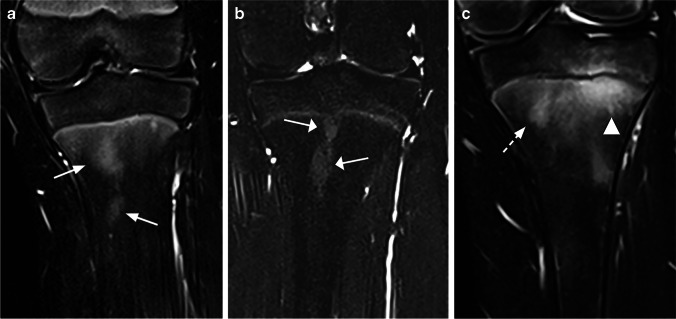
Coronal water-only Dixon T2-weighted magnetic resonance images in (a) a 10-year-old boy with an area of increased signal intensity in the metaphysis of the tibia with a diffuse contour (*arrows*), (b) a 16-year-old girl with an area of increased signal intensity in the metaphysis of the tibia with a sharp contour (*arrows*) and (c) a 14-year-old boy with an area of increased signal intensity in the metaphysis of the tibia with both a sharp and a diffuse contour (*dotted arrow* and *arrowhead*, respectively)
